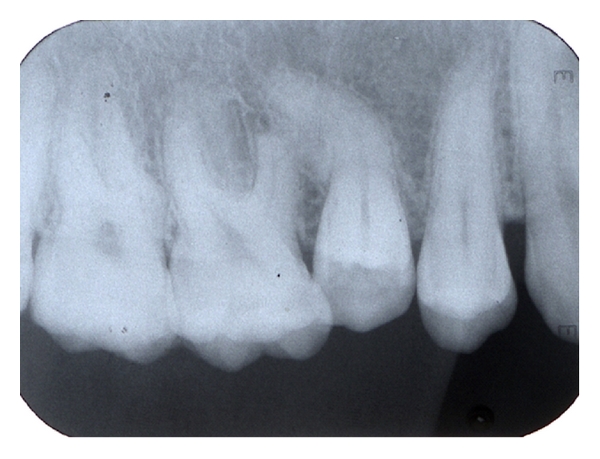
Initial periapical radiograph showing external apical root resorption resulted from the severely curved root of the adjacent premolar.
